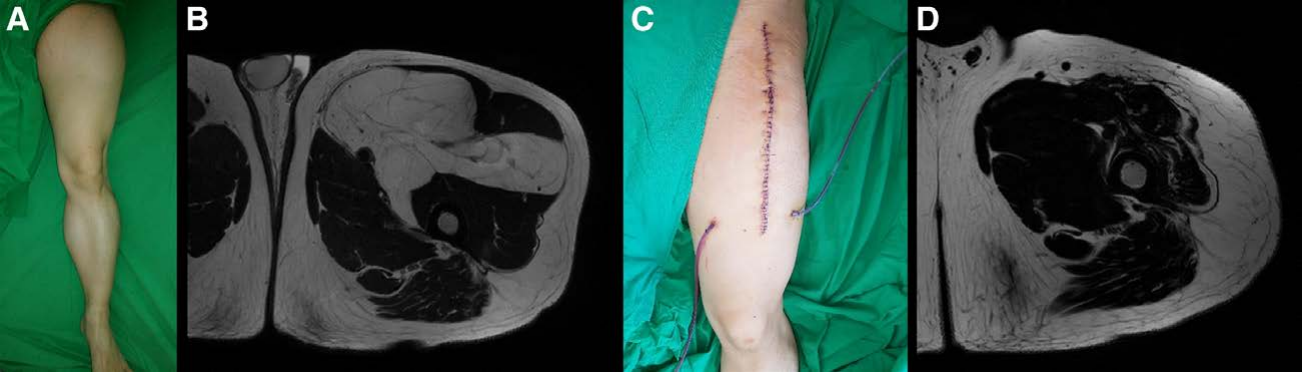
Case 1. M/44. (A) Preoperative clinical photo of the left leg. (B) Preoperative MRI, axial view. (C) Postoperative clinical photo of the left thigh. (D) Postoperative MRI, axial view. MRI, magnetic resonance imaging.
